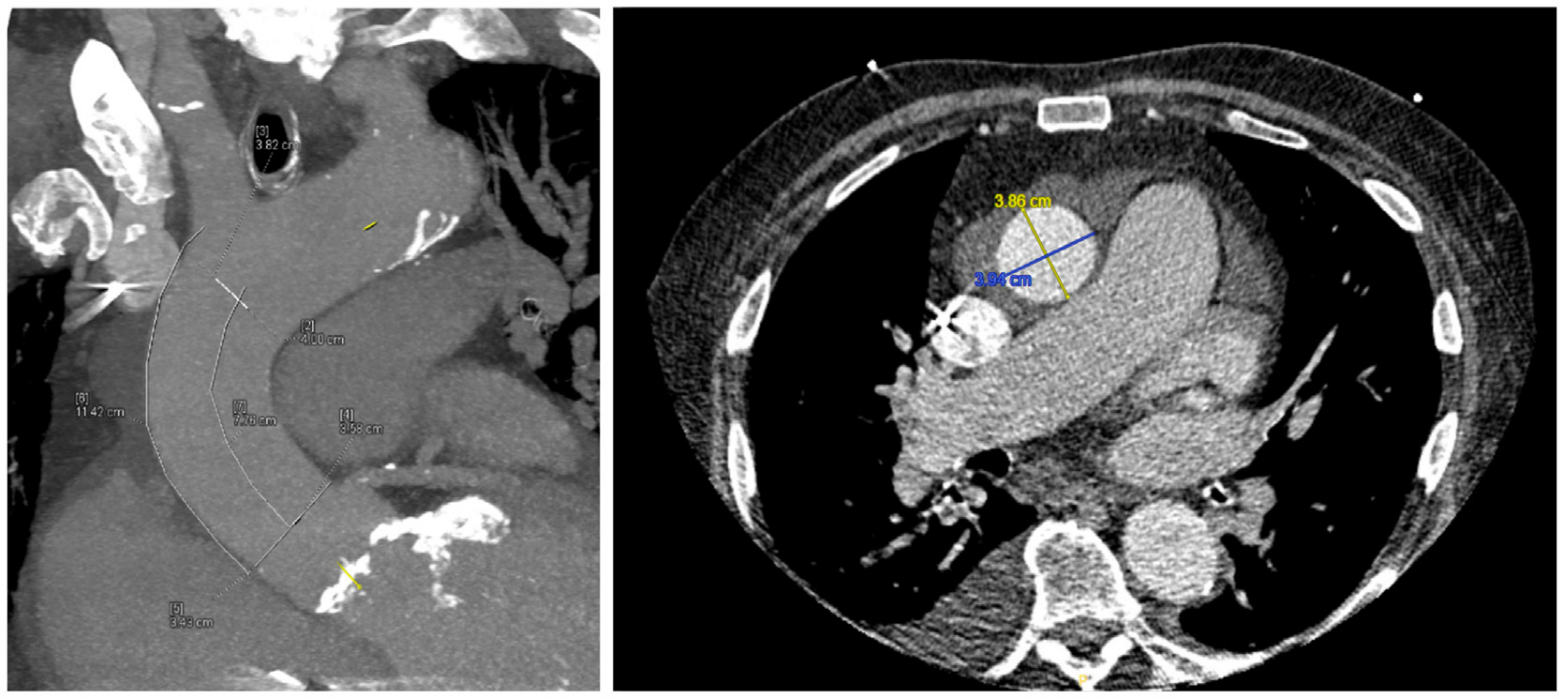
Preoperative computed tomography (CT) scan with labeled measurements of the ascending aorta.
